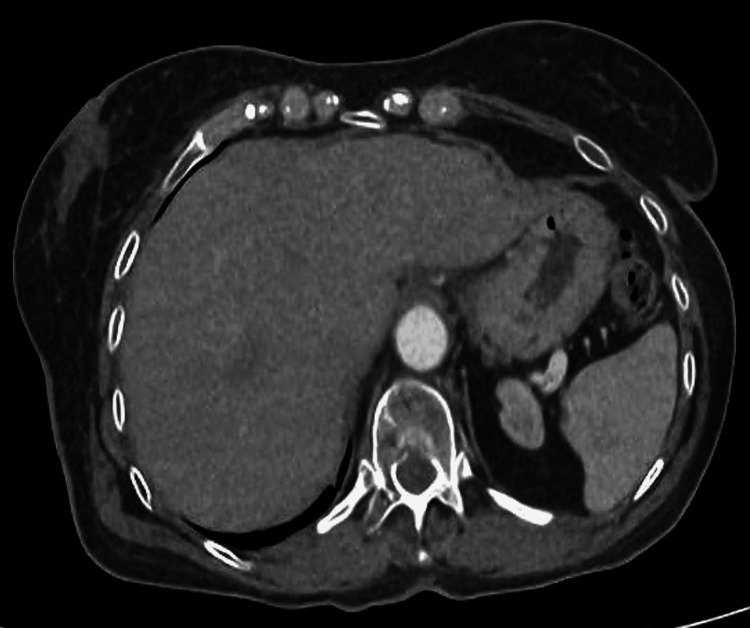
June 2024 CT scan showing complete response of hepatic lesions This image corresponds to the same plane as in Figure 3, with the disappearance of metastatic liver lesions after receiving 12 cycles of nivolumab.
